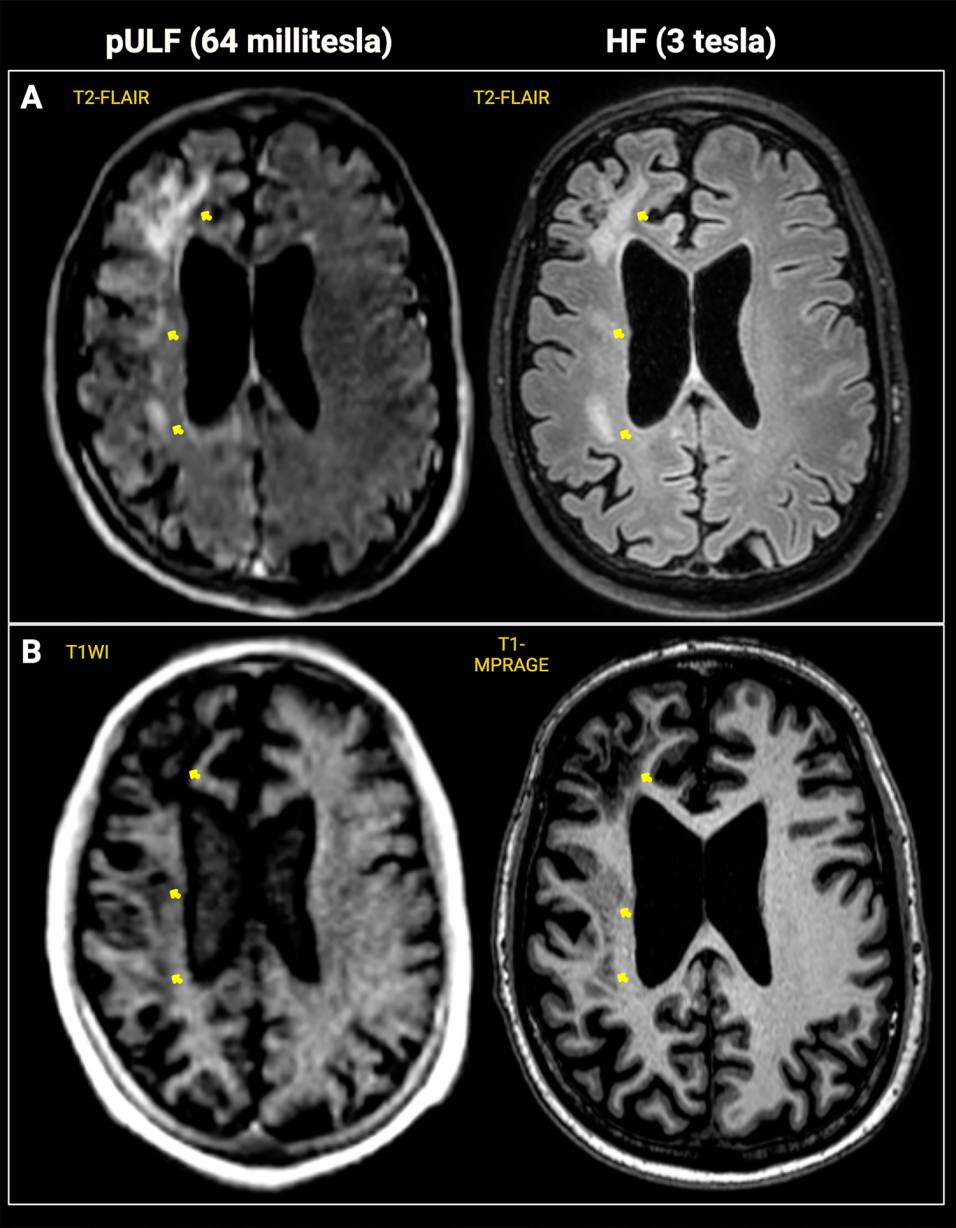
Representative image from a PML case showing pULF-MRI findings that correspond to signal characteristics of the PML lesion at HF-MRI. 61-year-old man with PML and underlying HIV. Extensive PML lesion in the right frontal white matter is visible on both pULF-MRI T2-FLAIR and HF-MRI (3 T) T2-FLAIR images (panel A) and both pULF-MRI T1WI and HF-MRI T1-MPRAGE images (panel B) with hyperintense and hypointense signal changes, respectively (yellow arrows)
